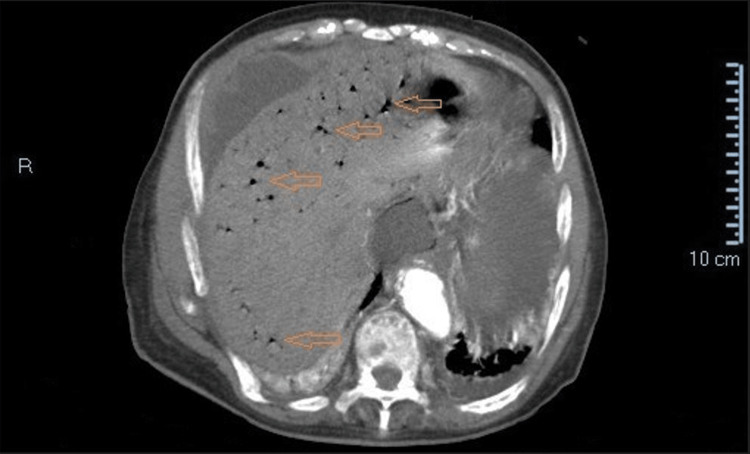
A contrast-enhanced CT scan shows exuberant signs of aeroportia (orange arrows)
